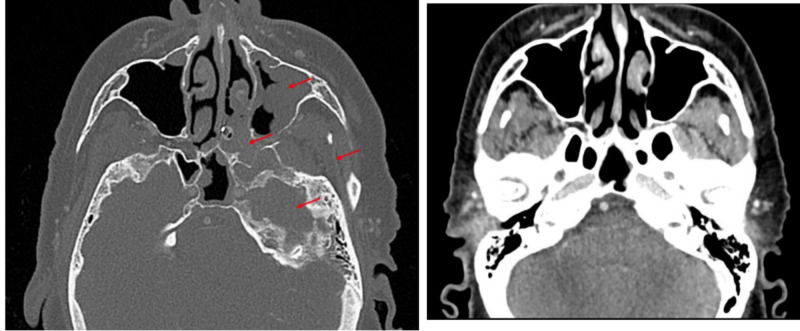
Case two computed tomography Left: Red arrows denote mass; Right: After two months of treatment
